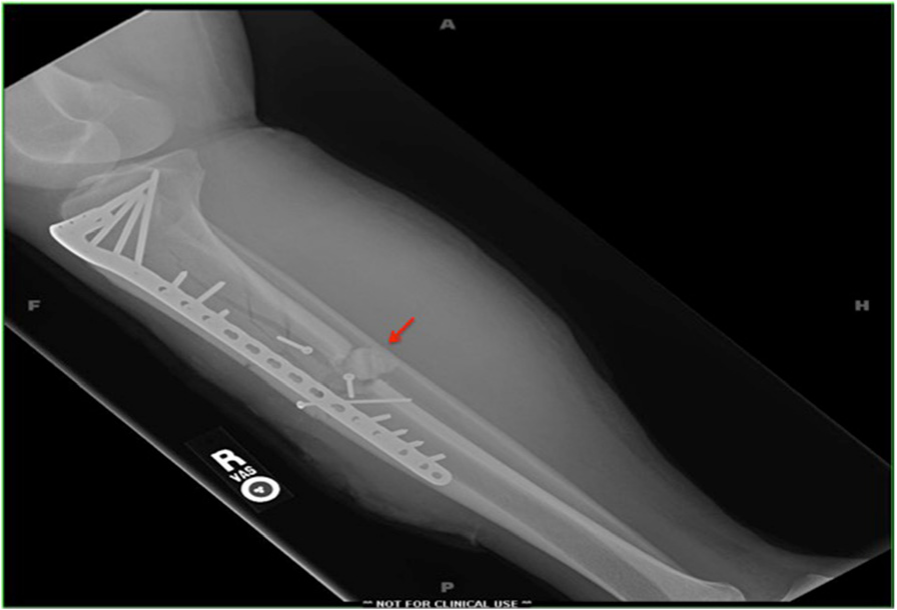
Preoperative lateral radiograph demonstrating a mid-diaphyseal comminuted fracture of the right tibia and sequestrum (red arrow)
